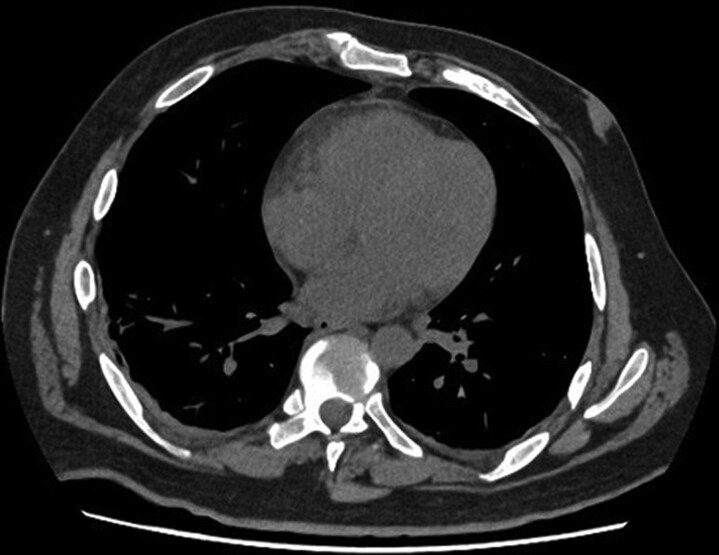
Baseline non-contrast chest CT on day 11 of hospitalization. Axial view showing no evidence of myocardial calcification. The left ventricular wall appears normal, without abnormal density or hyperattenuation.
